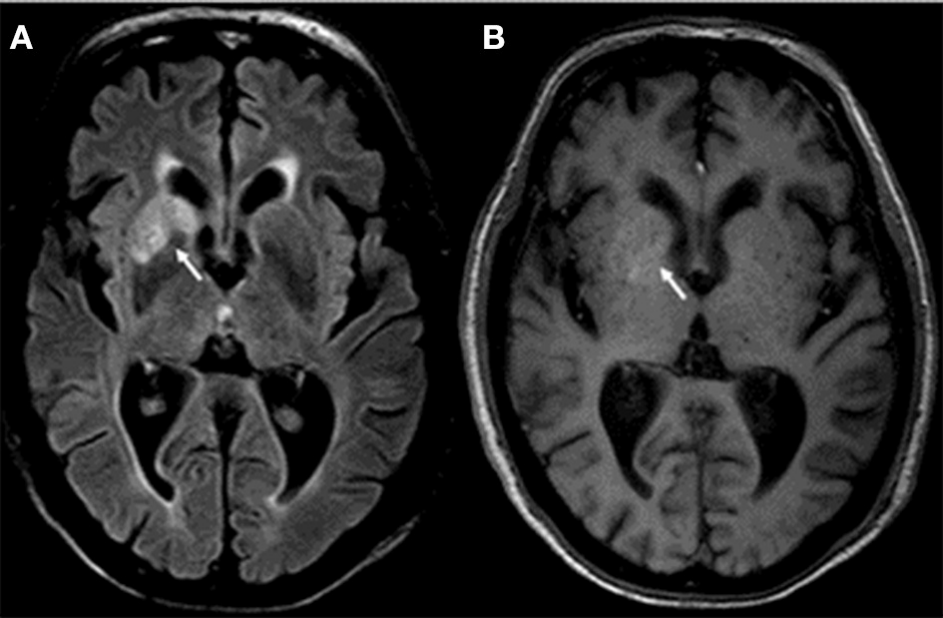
Brain MRI shows signal hyperintensity at the level of the basal nuclei (caudate nucleus head and putamen) on the right side in the FLAIR protocol (A). Discrete hyperintensity is also observed in the same region in the T1 protocol (B).
